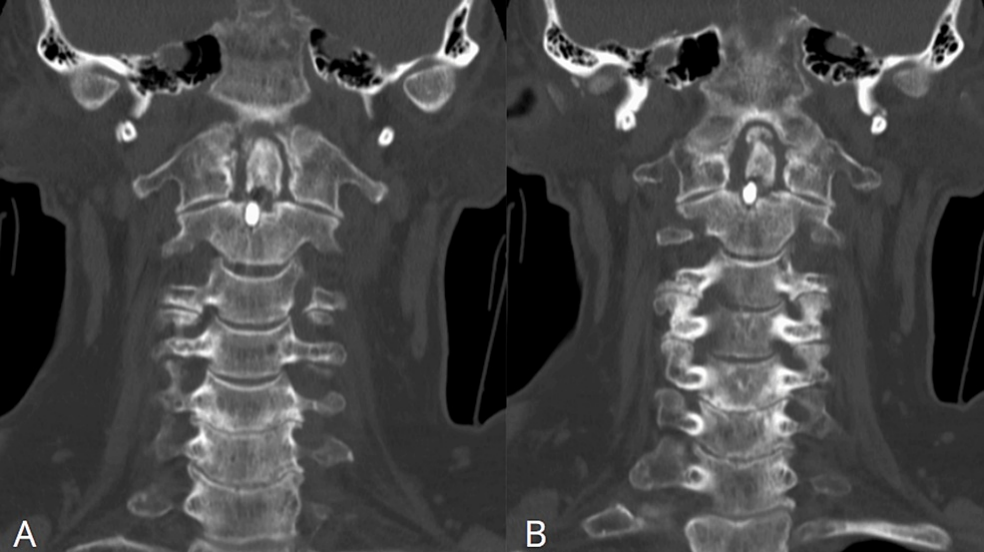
Cervical coronal CT Final positioning of the anterior odontoid screw (A). Good positioning of the anterior odontoid screw (B).
